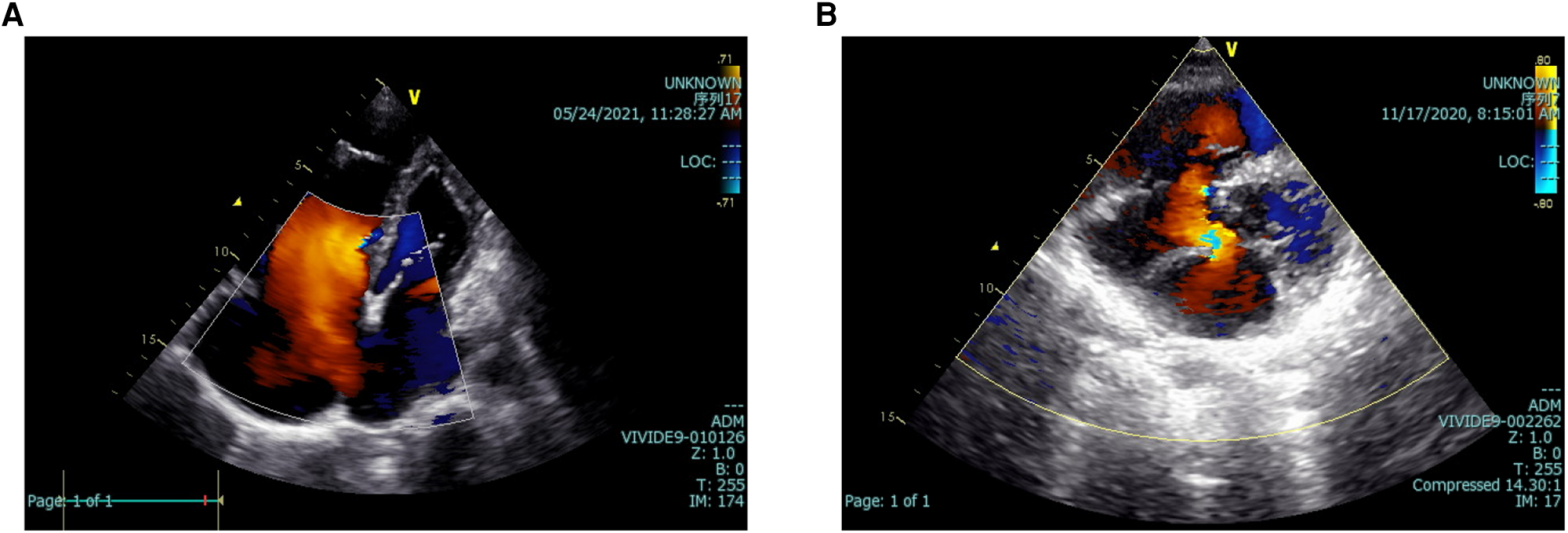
Echocardiograms of two kinds of ASD. The echocardiogram of secundum (A), and primum (B) ASD. Doppler ultrasound shows the direction and location of atrial shunting and the defect location. ASD, atrial septal defect.
